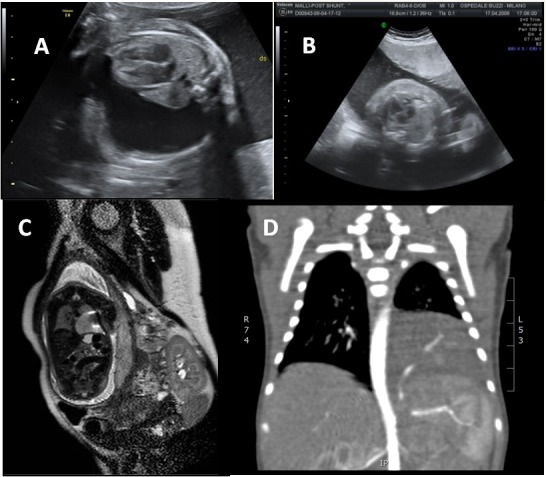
Figure 2: Case prenatally treated with thoraco-amniotic shunt positioning. Fetal US imaging of hydrothorax (A); reduction of fetal hydrothorax after TAS positioning (B: US imaging and C: fetal MRI); CT-scan at birth (D).
